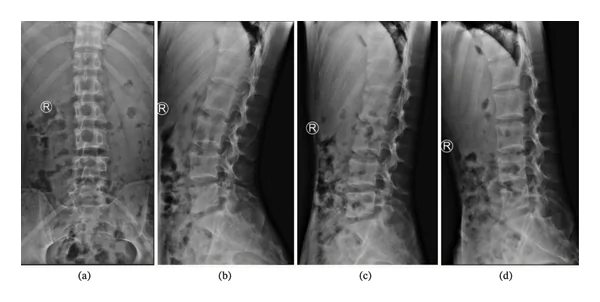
Preoperative lumbar radiographs. (a) Anteroposterior view; (b) lateral view; (c) hyperextension view; and (d) hyperflexion view.
